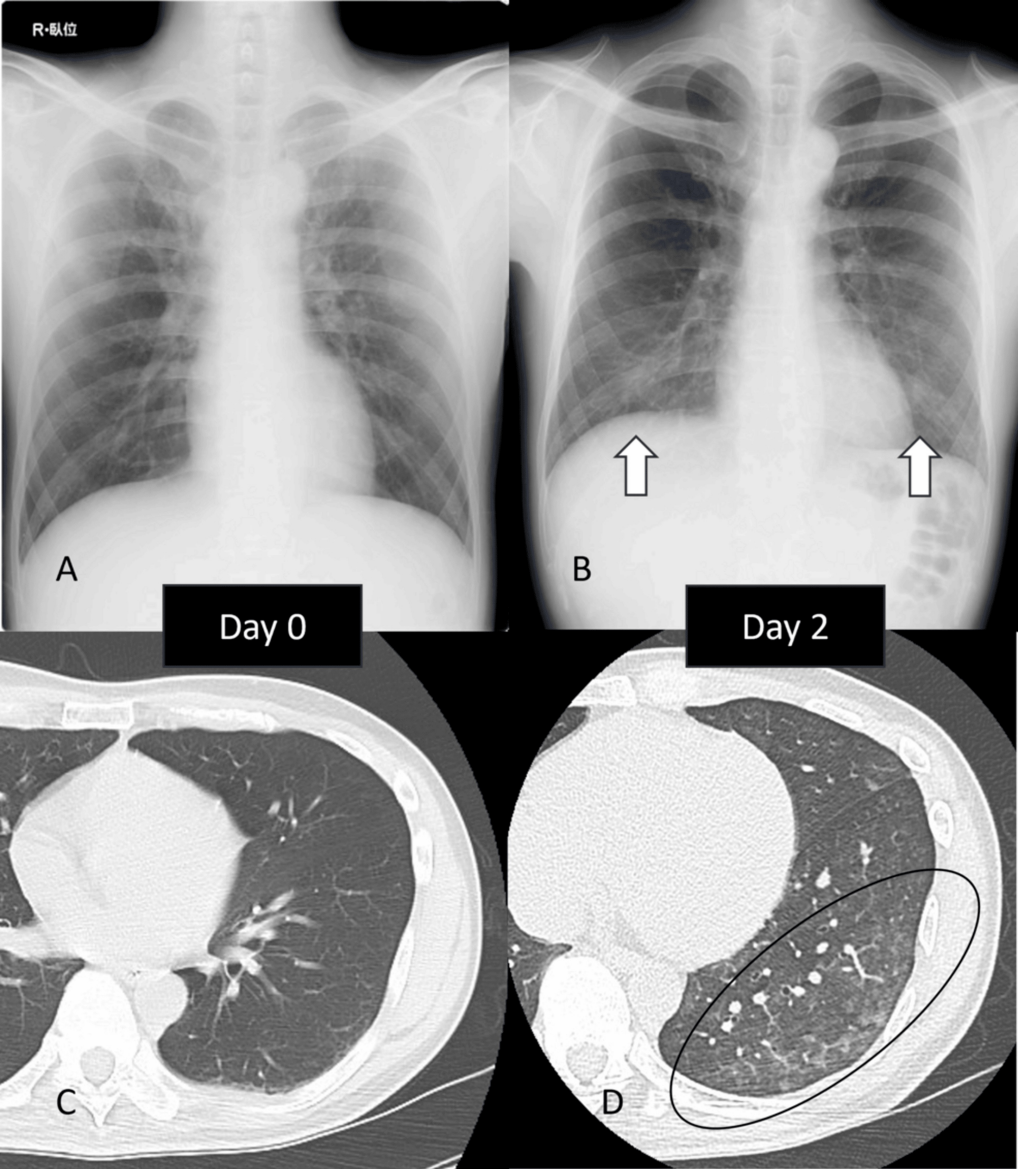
Radiographic and HRCT findings of nitrogen oxide inhalation injury (A) Chest radiograph on Day 0 showing no abnormalities.
